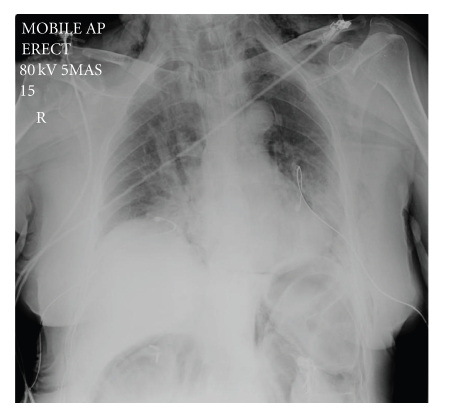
X-Ray post chest drain insertion. Note the presence of extraluminal air in the abdomen.
